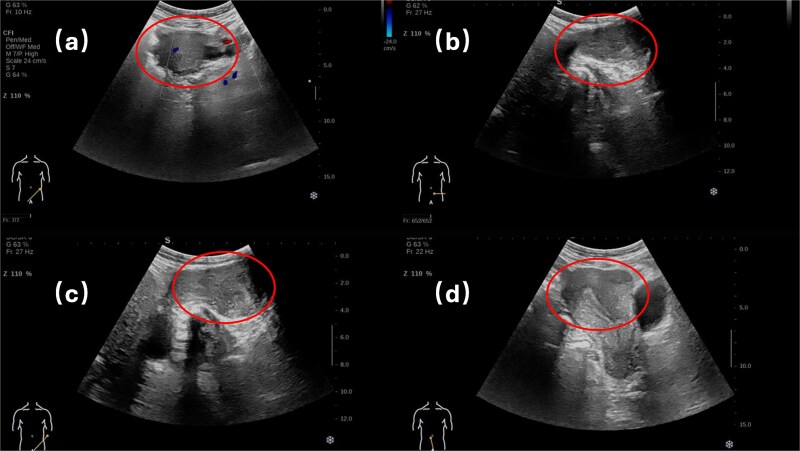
Superficial organ ultrasound and transvaginal ultrasound.
